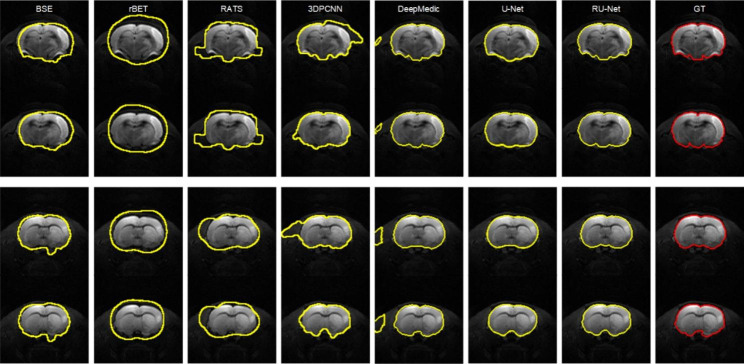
Visual comparison of DWI skull stripping results using different methods. Top row: slices 7 and 8 of Subject 10. Bottom row: slices 9 and 10 of Subject 21
